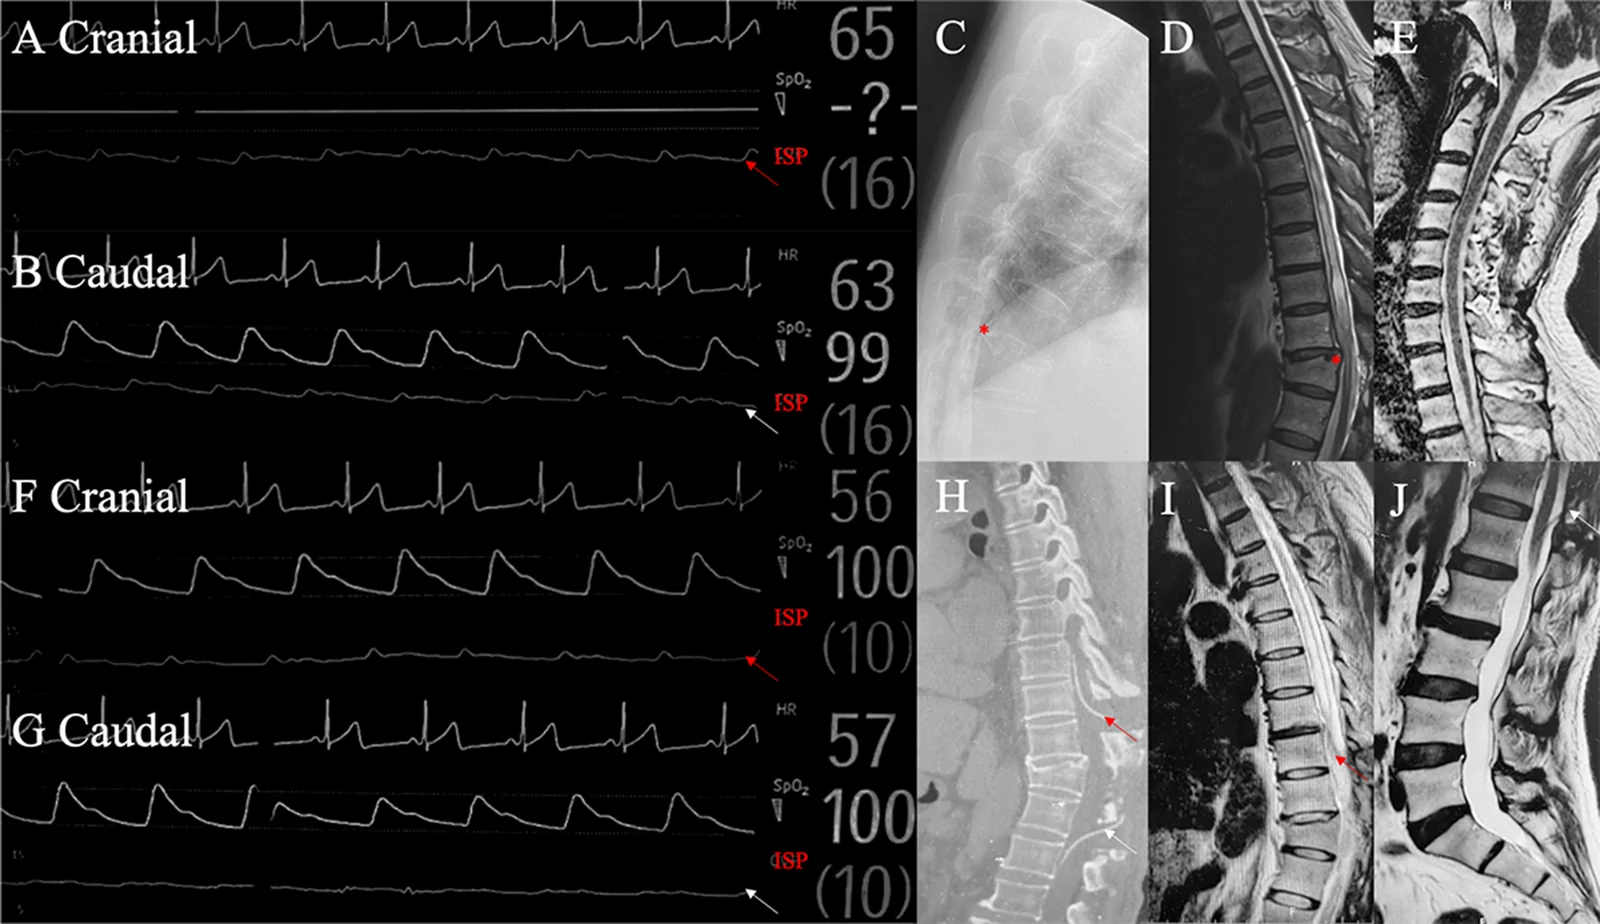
A 60-year-old male presented with progressive leg motor decline over two years, two decades after sustaining a T11-level SCI in a traffic accident. CASE3: (A, B) Preoperative intraspinal pressure (ISP) tracings cranial (A, red arrow) and caudal (B, white arrow) to the arachnoid adhesion at T11 show no abnormal pulsatile ISP wave. (C) Lumbar myelogram confirming a complete subarachnoid block at the T11 level (red asterisk) corresponding to dorsal arachnoid scarring.(D, E) Preoperative T2-weighted magnetic resonance (MR) images demonstrate an intramedullary syrinx extending rostrally to T5. (F, G) Postoperative ISP tracings at corresponding locations (F: cranial, G: caudal) following S-S bypass placement show stable waveforms without a significant change in the pressure gradient. (H) Postoperative computed tomography (CT) localizer image confirming the position of the S-S bypass conduit spanning from T10 (red arrow) to L1 (white arrow). (I, J) Postoperative T2-weighted MR images showed no change in the syrinx morphology
